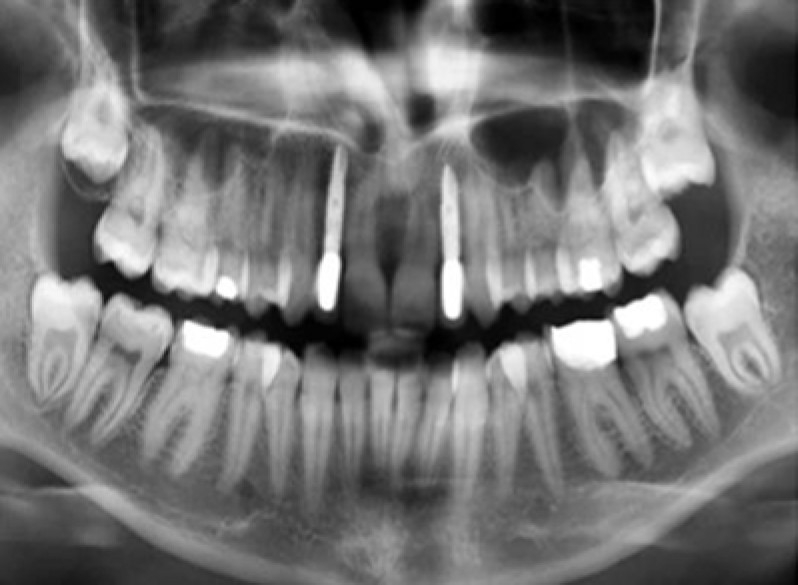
Panoramic radiograph taken after two years.
